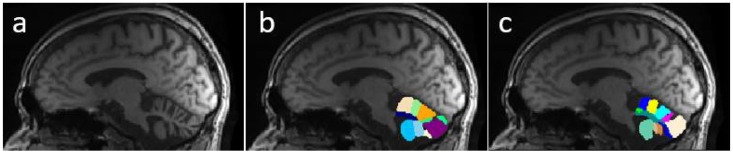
Comparison between segmentations on MRI of SCA2 patient. In (a) the original imaging, in (b) segmentation produced by ACAPULCO, and in (c) segmentation obtained by CERES.
